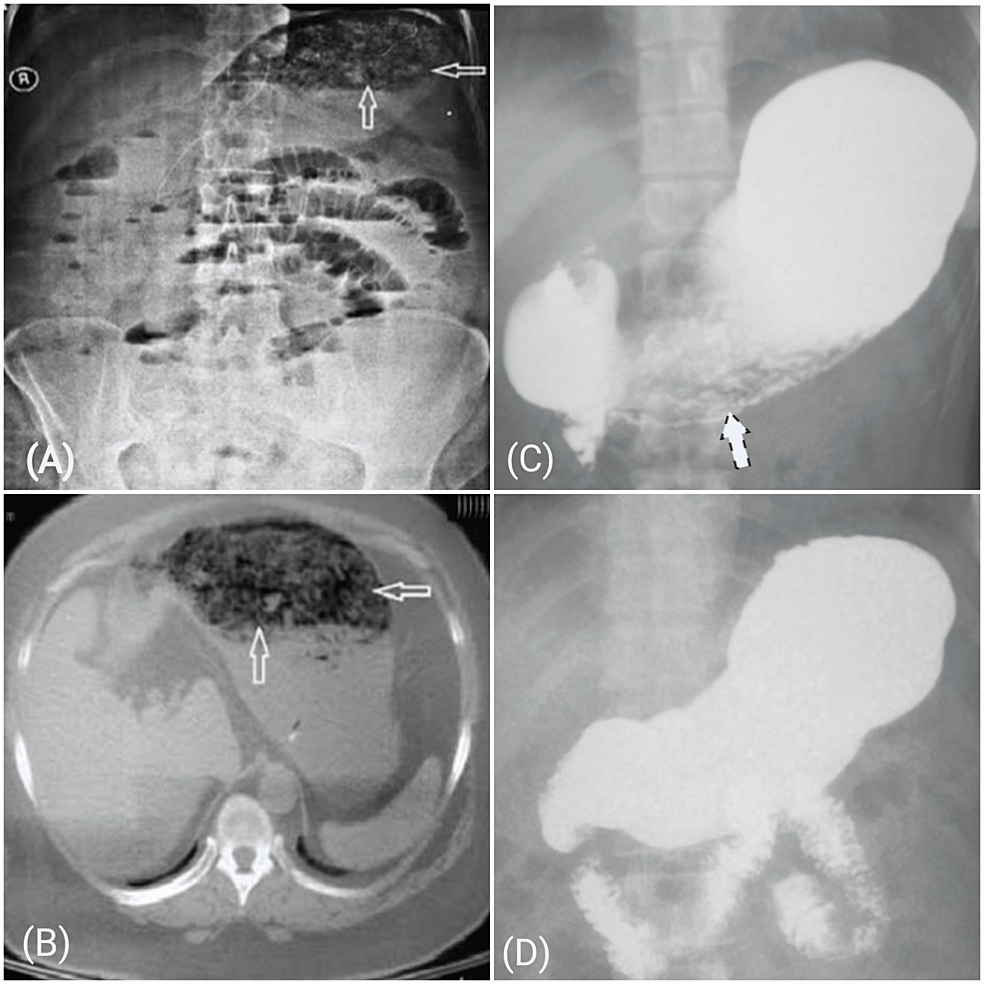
Erect abdomen x-ray (A) shows a shadow in the stomach (black arrows) and distended distal bowel; CT scan (B) shows a characteristic mottled appearance in the stomach; barium meal shows filling defect (C); and barium meal shows no filling defect and free passage of contrast in distal bowel (D) in a patient diagnosed with gastric outlet obstruction secondary to persimmon-related gastric phytobezoar before (A)-(C) and after (D) intervention. CT: computed tomography.
